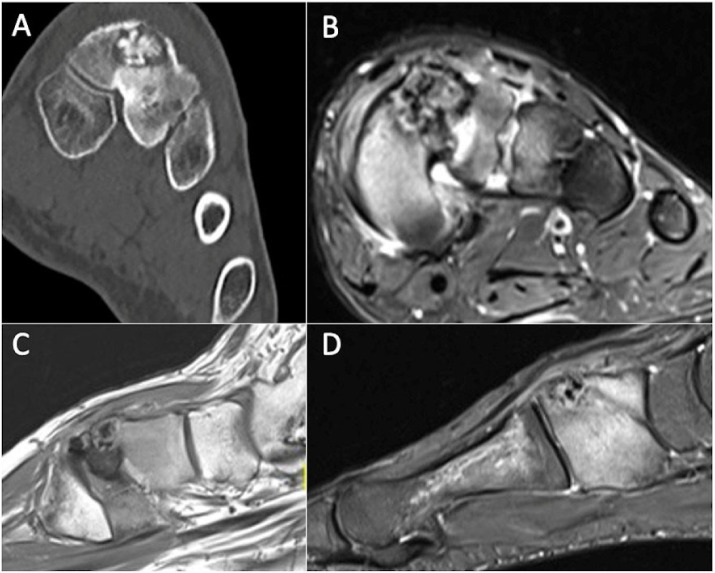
Coronal CT (A) and STIR MRI (B) demonstrating an osteoblastoma of the medial cuneiform, with marked surrounding sclerosis and both osseous and soft tissue oedema, larger than the size of the lesion itself. Sagittal T1-weighted (C) and STIR (D) coronal MRI demonstrate the oedema involving almost the entire medial cuneiform.
